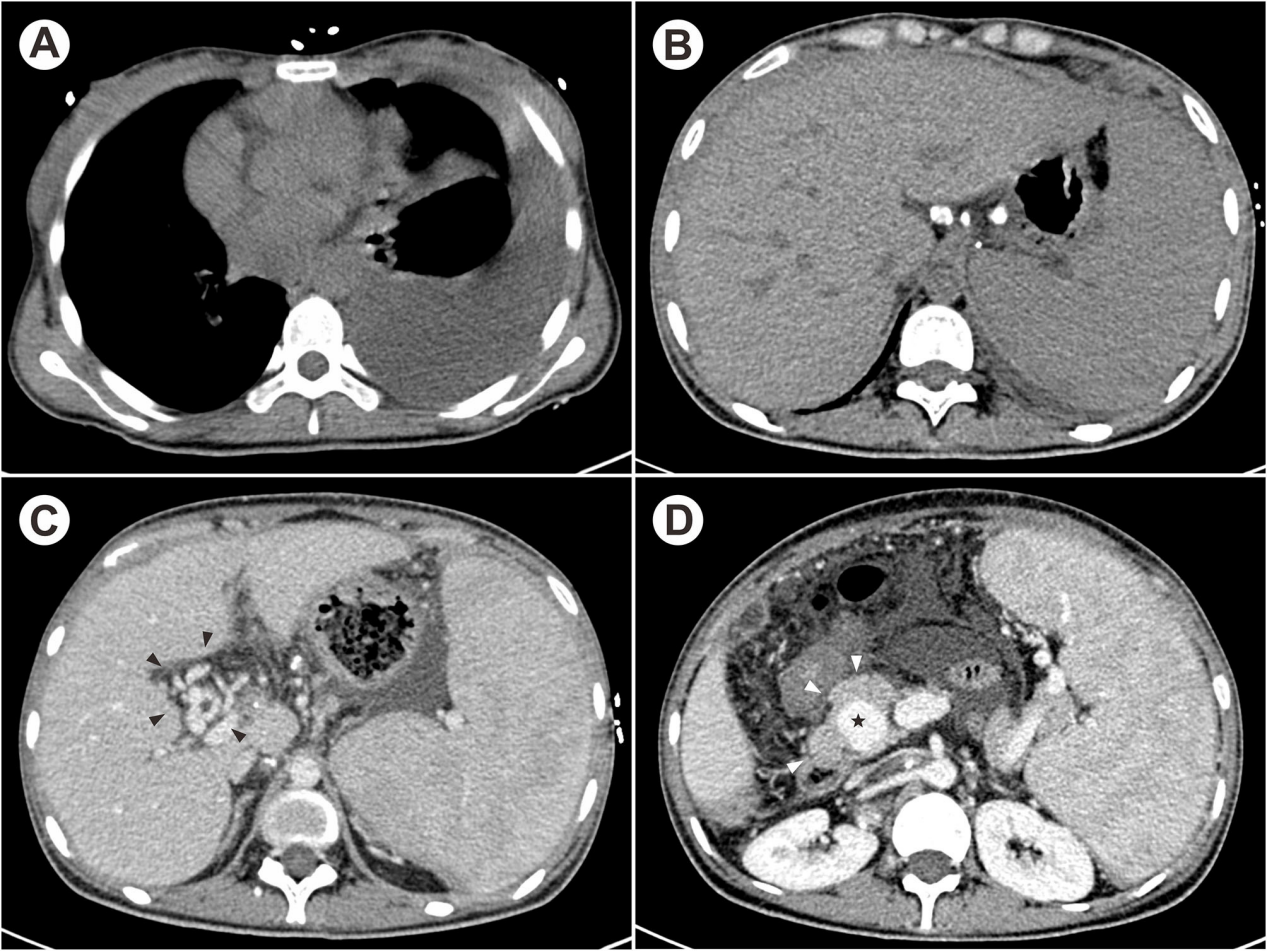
The contrast-enhanced CT showed massive pleural effusion on the left chest (A), multiple calcified hilar lymph nodes (B), CTPV [(C), black arrowheads] and distal portal venous aneurysm [(D), star] due to lymphadenopathy [(D), white arrowheads].
